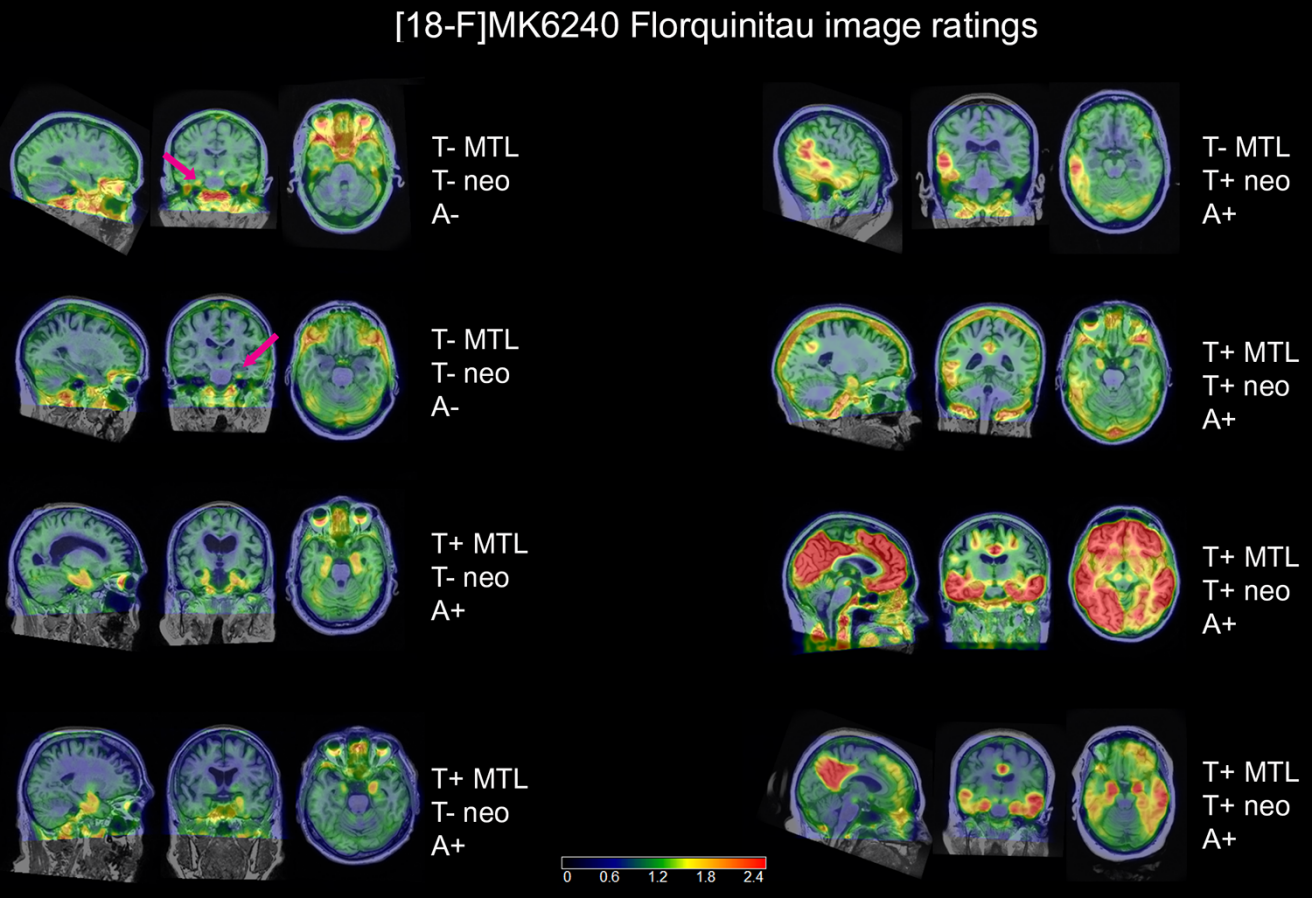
Examples of FQT PET exams in each of the tau rating categories. In each case, the standardized uptake value ratio is overlaid on a co‐registered T1‐weighted MRI and is shown in sagittal, coronal, and axial views at informative slice locations. The arrow in the first two cases points to an area of subtle punctate MTL signal that was considered tau negative. The ratings for tau PET in the MTL and neo and for amyloid PET are shown to the right of each case. FQT, [F‐18]florquinitau; MRI, magnetic resonance imaging; MTL, medial temporal lobe; neo, neocortex; PET, positron emission tomography.
